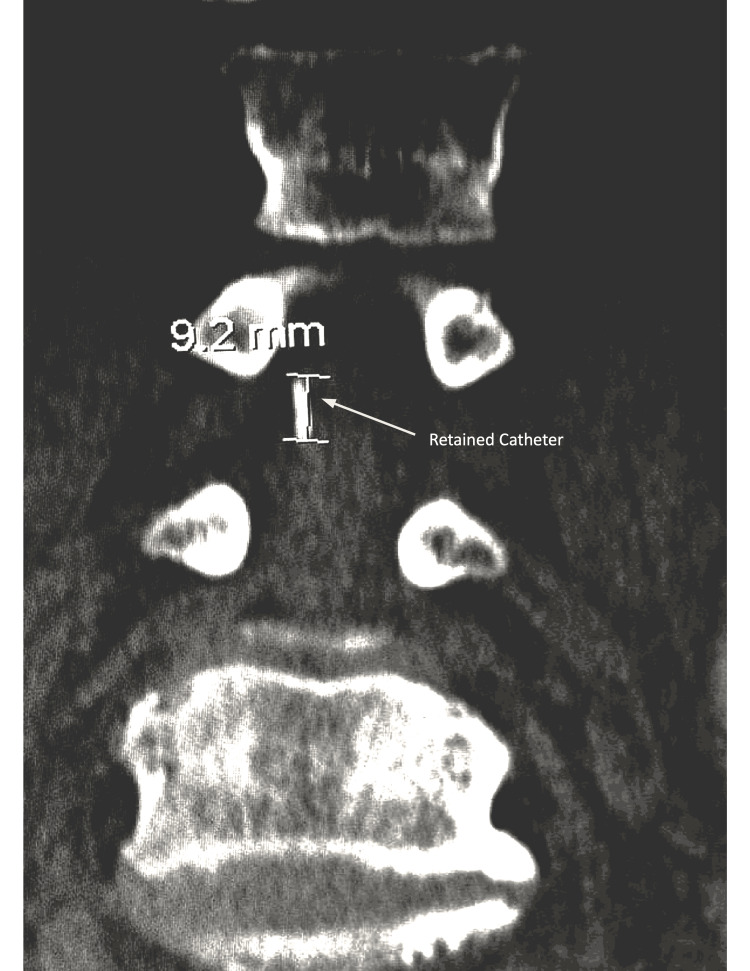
Lumbar spine CT Coronal view. The arrow indicates the retained catheter fragment.
